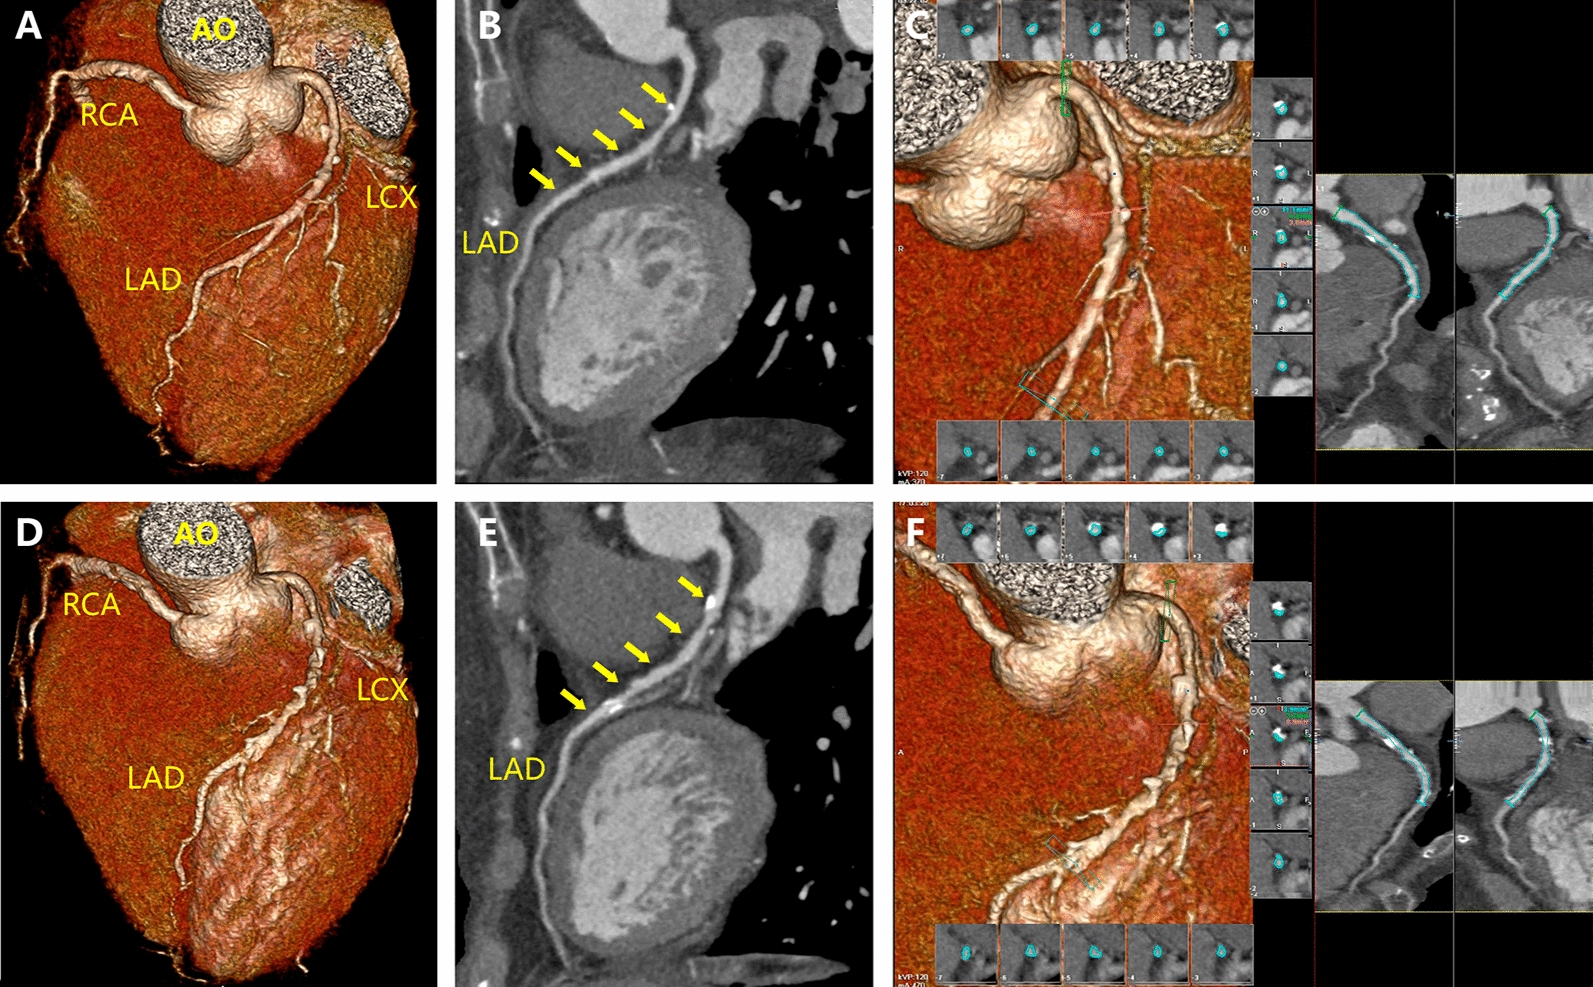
Representative imaging for the change of the total plaque volume at the beginning and the follow-up. The 3-dimensional reconstruction and analysis of coronary CTA images of a 60-year-old female patient with type 2 diabetes were performed. The baseline CTA showed a moderate stenosis in the proximal segment of the left anterior descending coronary artery (a, b), with a total plaque volume of 119 mm^3^ (c). After a 2.8-year follow up, clinically significant progression (d–f) was observed, with the total plaque volume progressed to 194 mm^3^. The annual progression rate in total plaque volume was 22.51%
